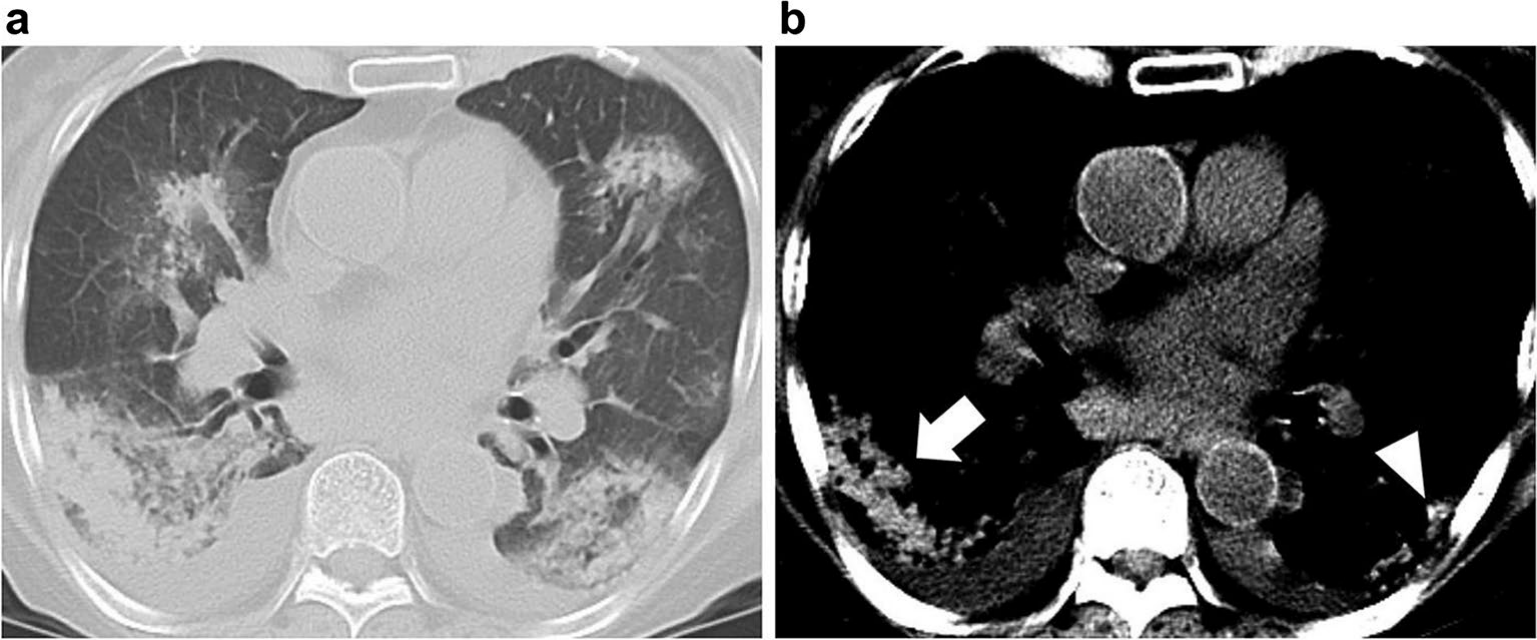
78-year-old woman with diffuse alveolar hemorrhage in Sjögren’s syndrome. (a) Transaxial CT with a lung window setting (window level =  − 600 HU, width = 1600 HU) showing consolidations in both lungs. (b) In this study, the high-density area in the right lung, which is obviously higher than that of the aorta in the narrow window setting (window level = 35 HU, width = 80 HU), is interpreted as the hyperdense consolidation sign (arrow). On the other hand, the small nodular high-density lesion in the left lung, which can be confused with calcification or artifacts, is interpreted as NOT showing the hyperdense consolidation sign (arrowhead).
